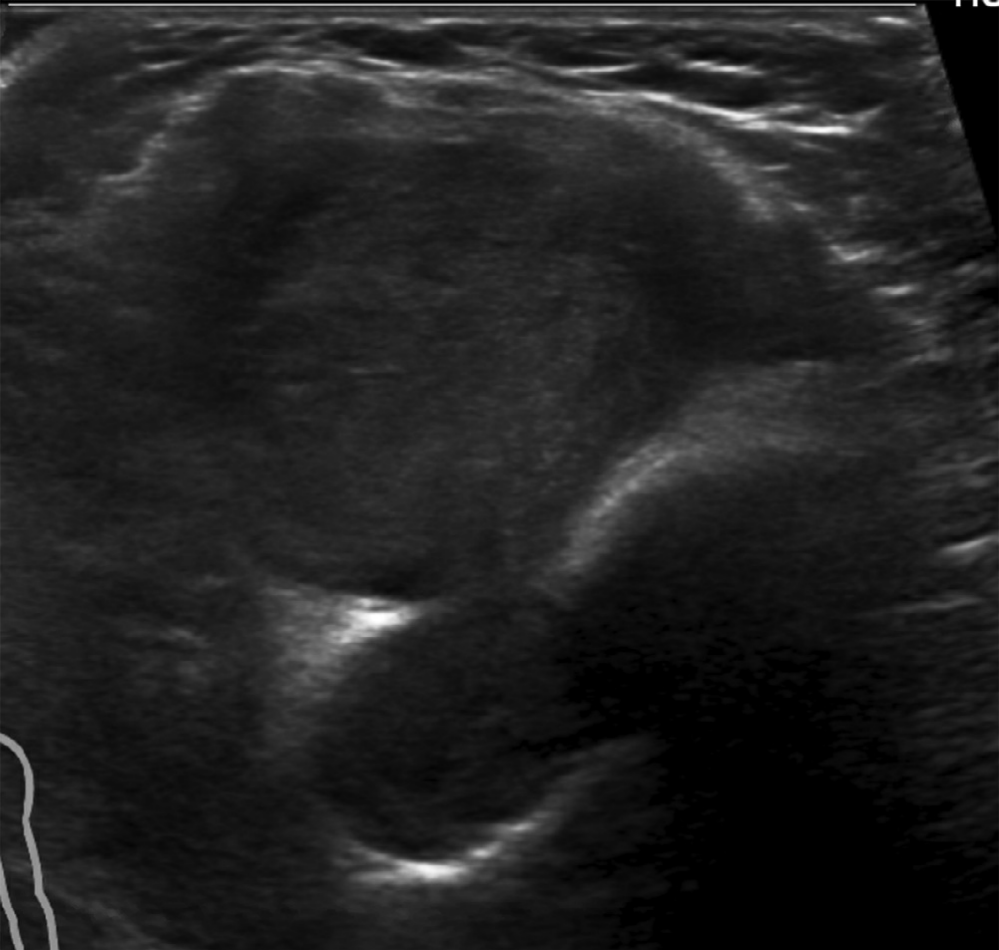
Transversely oriented ultrasound of the right antecubital region demonstrating a soft tissue mass involving the anterior aspect of the humerus. The center of the mass is isoechoic, and the periphery is hypoechoic.
